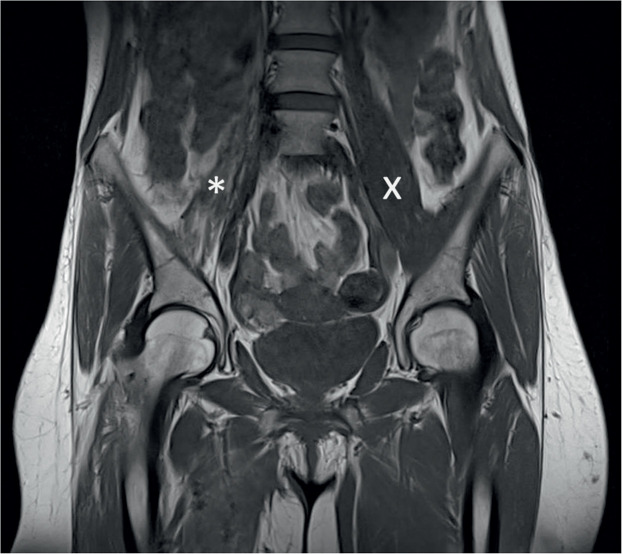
Atrophic psoas muscle (*) compared to the uninjured contralateral muscle (x) in a MRI scan 6 months after tendon release.
